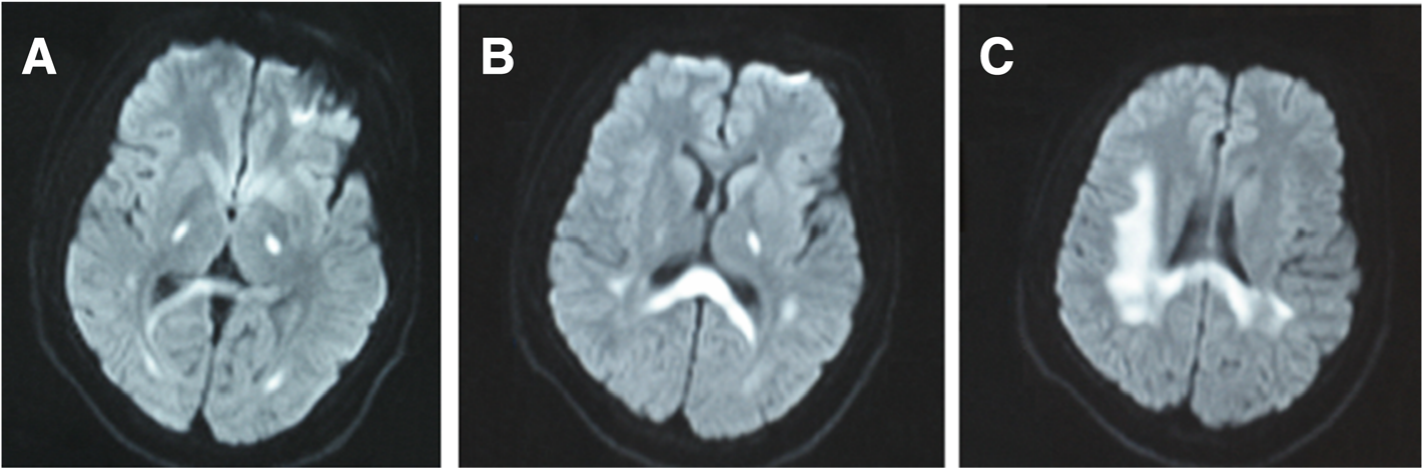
The first MRI after four days of onset. Diffusion-weighted MRI after four days of the onset of symptoms, which presents repeated transient weakness of the limbs, slurred speech and dysphagia, shows diffuse signal abnormalities in the internal capsule (A and B), corpus callosum (B) and periventricular areas (C). Neurologic examination reveals musclar atrophy of distal limbs, diminished deep tendon reflexes in all extremities and giving the characteristic inverted champagne bottle appearance.
